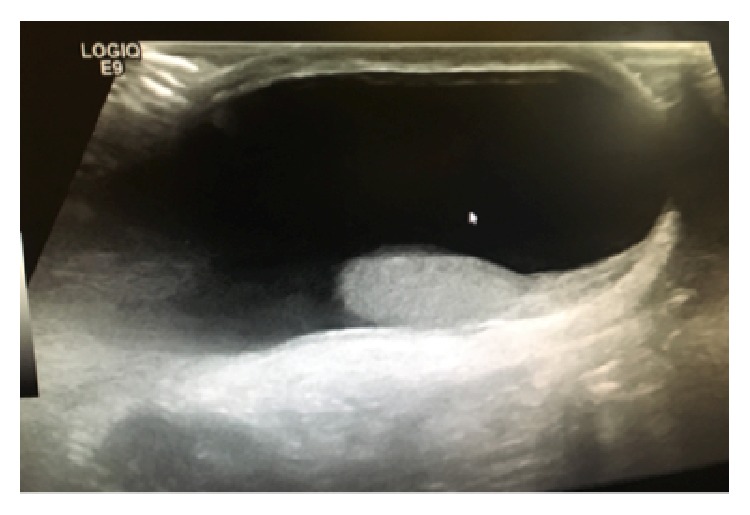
Ultrasound examination. Sagittal image of the left scrotal mass demonstrating the difficulty to appreciate the full extent of the abnormality.
